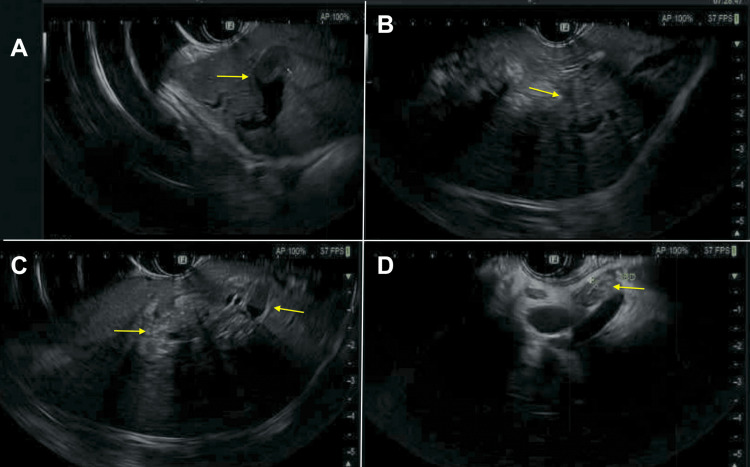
Follow-up EUS images (A-C) There is persistence in abnormal intrahepatic bile duct dilation involving the lateral greater than medial left lobes of the liver with small amounts of pneumobilia in the left liver lobe with indistinct mass centrally within the left lobe of the liver measuring approximately 2.2 x 1.8 cm (yellow arrows). (D) Common bile duct with dilation (yellow arrow). EUS: endoscopic ultrasound.
